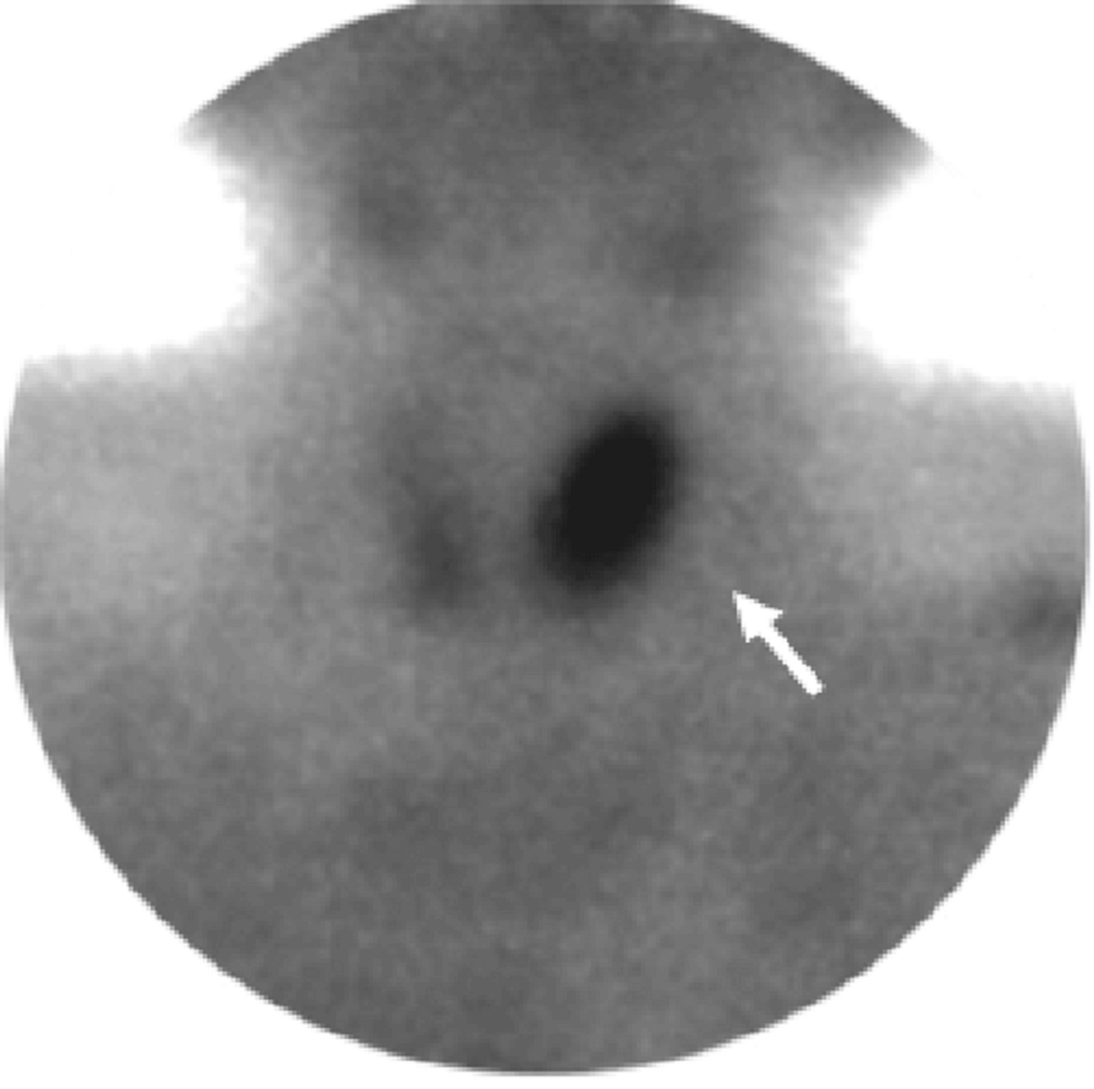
99mTecnecium methoxy-isobutyl-isonitrile (MIBI) scintigraphy 99mTecnecium MIBI scintigraphy demonstrated uptake in the same location on the left thyroid lobe. Tumor indicated by the white arrow.
